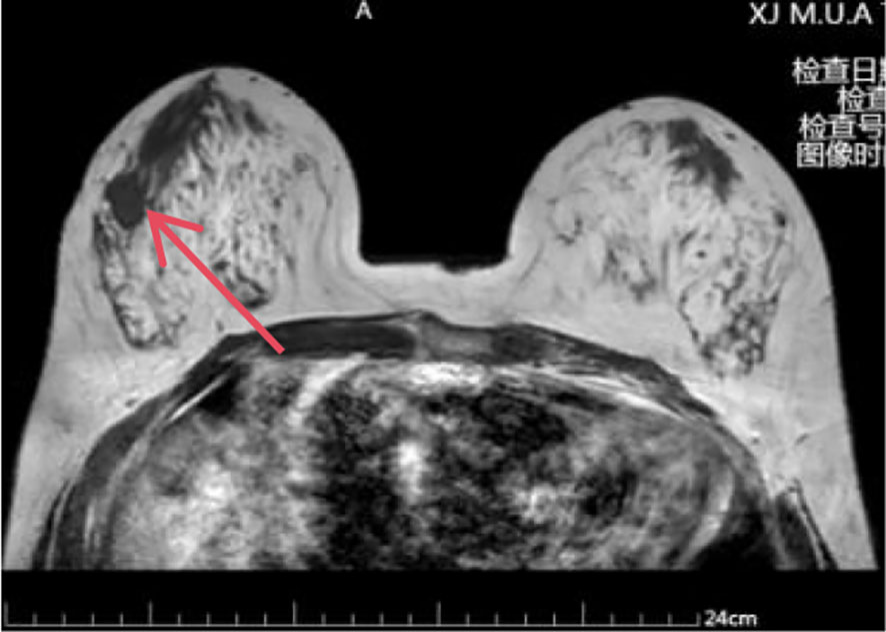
MRI: A round mass measuring approximately 1.9 × 1.4 × 1.7 cm is visible in the right breast’s outer lower quadrant.(The red arrow in the image points to the mass.).
